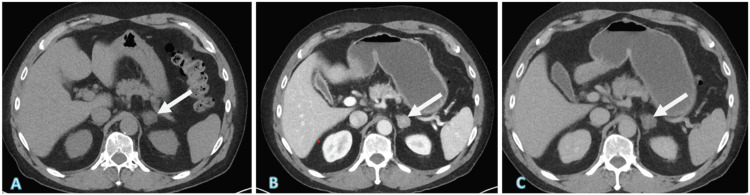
Left adrenal adenoma: axial CECT adrenal protocol images demonstrate a circumscribed left adrenal nodule (white arrows) displaying low attenuation on the non-contrast scan (A), post-contrast enhancement on the venous phase image (B), and significant delayed washout on subsequent imaging (C), characteristic of a lipid-rich adrenal adenoma. CECT = contrast-enhanced CT
